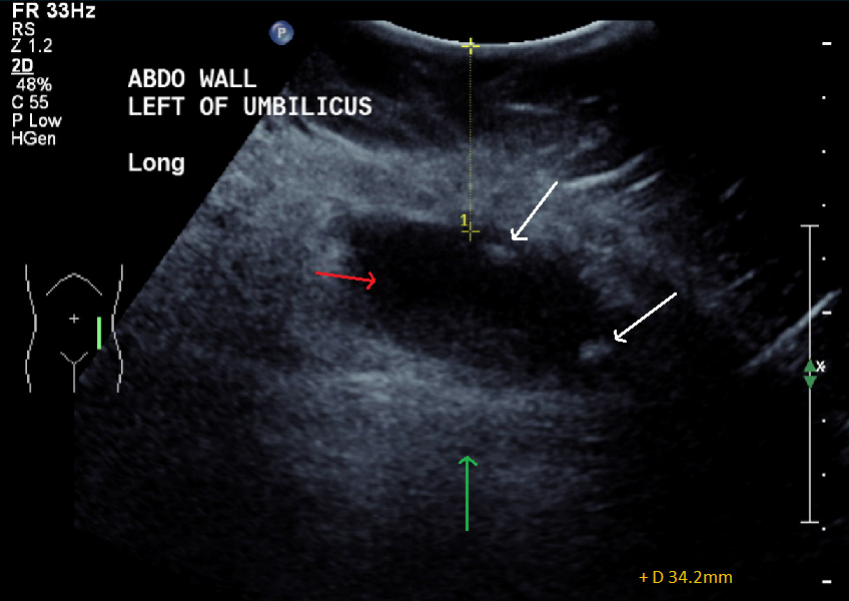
Operative planning ultrasound prior to ultrasound-guided subcutaneous
